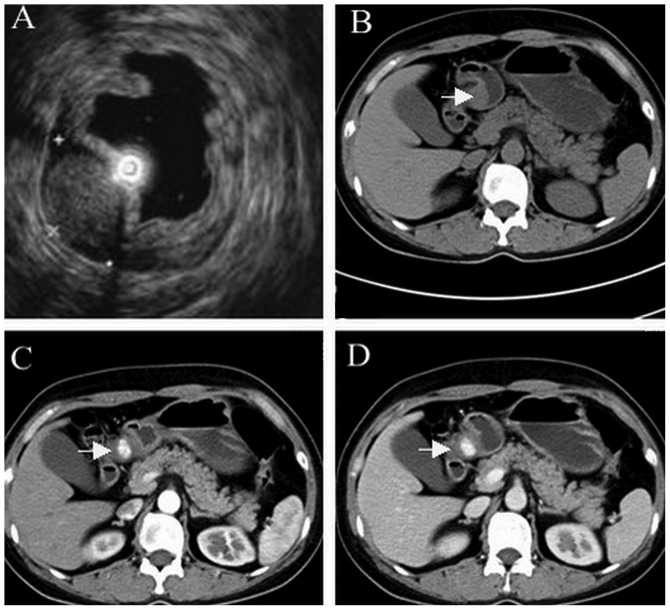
(A) EUS an ovoid, demarcated, heterogeneous, hyperechoic tumor 18.6×11.8 mm in size, originating from the fourth EUS layer (muscularis propria). Abdominal computed tomography revealed a well-demarcated, ovoid mass at the antrum (arrow) on (B) unenhanced, (C) arterial- and (D) delayed-phase scans. EUS, endoscopic ultrasound.
